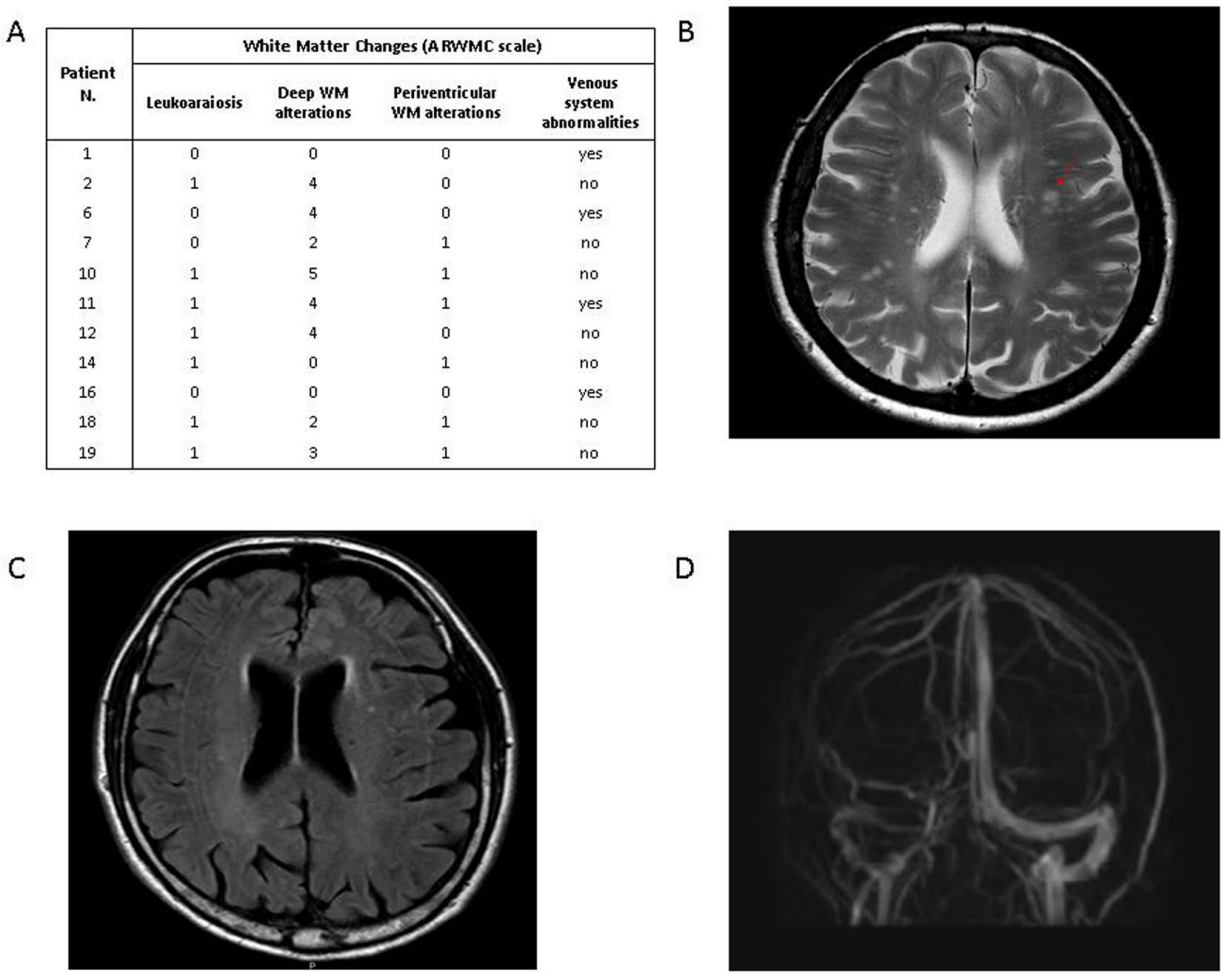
Pathological brain MRI findings in PNH patients. (A) White Matter Changes (ARWMC scale, see Materials and Methods) (B). The T2 weighted axial image shows several t2 focal hyperintensities of WM due to chronic microinfarcts (patient N.10); (C) The FLAIR axial image shows periventricular hyperintensity due to vascular chronic degeneration (patient N. 7); (D) The Phase Contrast acquisition shows the asymmetry of venous sinuses with irregular walls of the right transverse sinus that can be due to a previously occurred venous thrombosis (patient N. 1). Control population (n = 38) findings: 9 leukoaraiosis, 19 deep WM focal chronic ischemic lesions (n = 5, score 1; n = 10, score 2; n = 3, score 3; n = 1, score 4); a lesion > 5mm was observed in 2 subjects aged 53 and 83 years.
